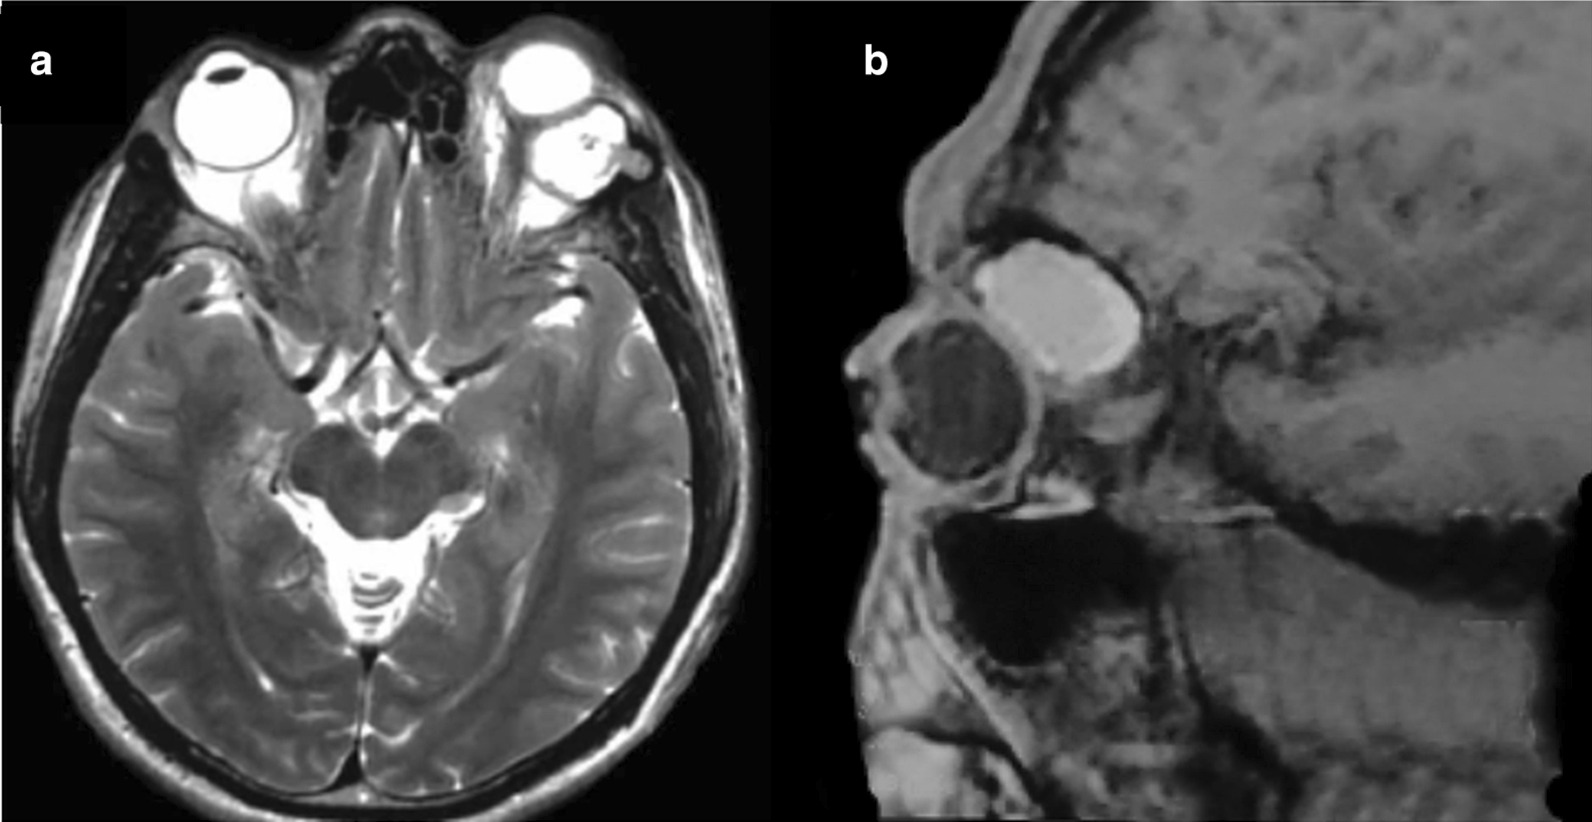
Preoperative clinical findings in a male patient with an orbital cholesteatoma. a Transversal T2-weighted magnetic resonance images (MRI) of the brain showing an hyperintense mass located at the superolateral orbital wall of the left eye. b Sagittal T2-weighted MRI which reveals the anterior displacement of the left eye and the invasion of the frontal bone caused by the tumor
